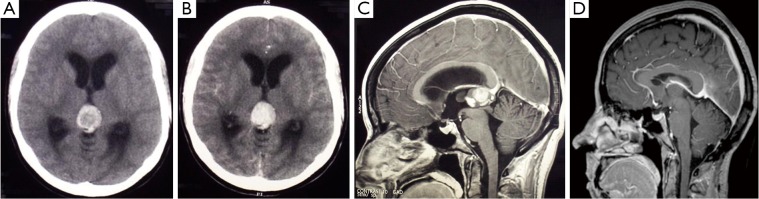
A. The plain CT scan shows a hyperdense pineal mass, which is suggestive of hemorrhage; B. The lesion is enhanced with contrast, and causes an obstructive hydrocephalus with the dilatation of the lateral ventricles. Sagittal T1-weighted gadolinium MRI scans; C. The pre-operative scan reveals a 2.0 cm × 2.5 cm pineal lesion with a well-defined margin, which compresses the cerebral aqueduct; D. The repeated scans at the 5-year follow-up shows no recurrence and complete tumor resection. The closeness of the internal cerebral vein and vein of Galen to the tumor should be noted. These veins remain obvious postoperatively. There is evidence of decompression of the ventricles and cerebral aqueduct.
